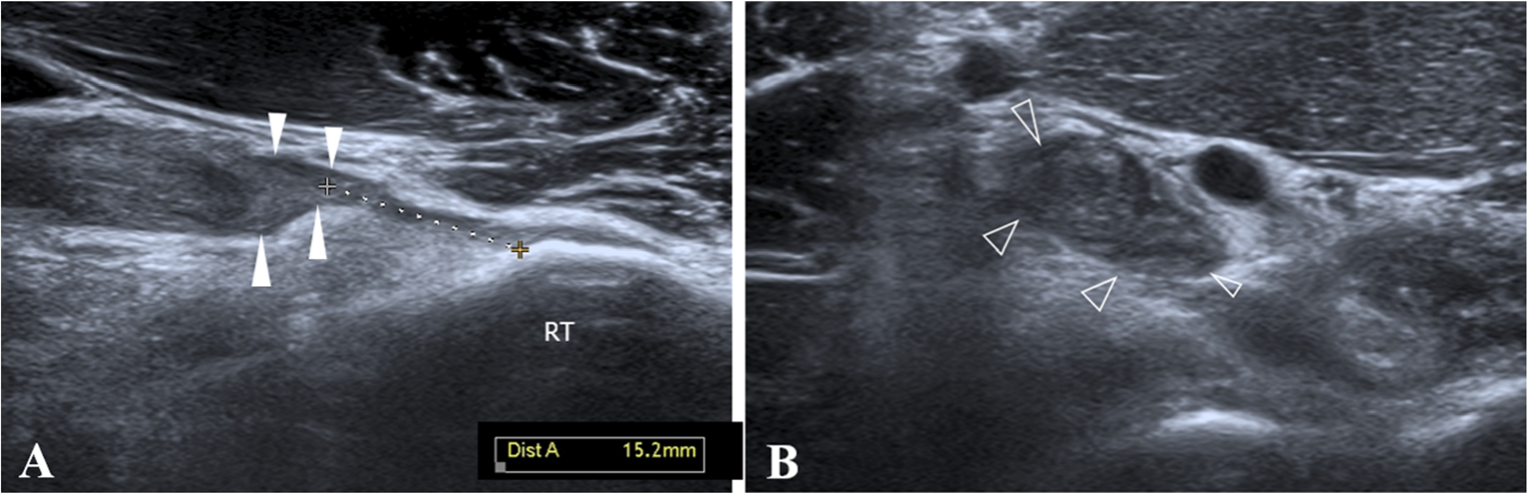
Ultrasound view of a type 3A injury (complete non-retracted tear due to intact EBA). a In the long-axis view, a DBBT tear with a mild retraction of the DBBT is observed and confirmed by measuring the gap between the stump (*white arrowheads*) and the radial tuberosity (*RT*). b Short-axis view of a. Cross-section of the DBBT stump (*arrowheads*) showing tendon hypertrophy and an altered echotexture
